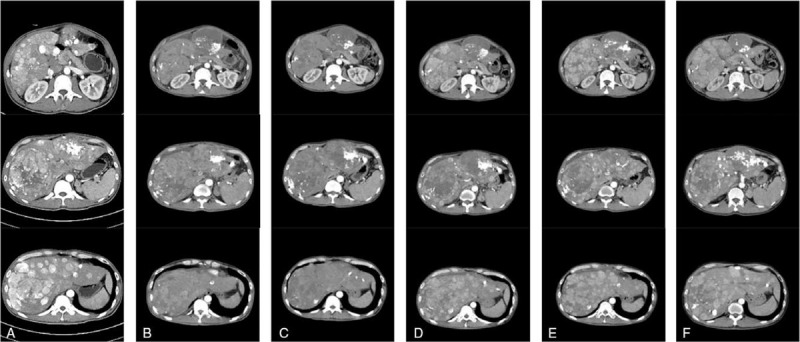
CT scan. (A) Baseline. CT scan showed partial response after lenvatinib plus pembrolizumab treatment for 1 month (B) and 3 months (C). (D) CT scan showed progressive disease after stopping lenvatinib. (E) CT scan showed progressive disease after treatment with nivolumab plus chemotherapy for 1 month. (F) CT scan showed stable disease after treatment with nivolumab plus olaparib for 3 months. CT = Computed tomography.
